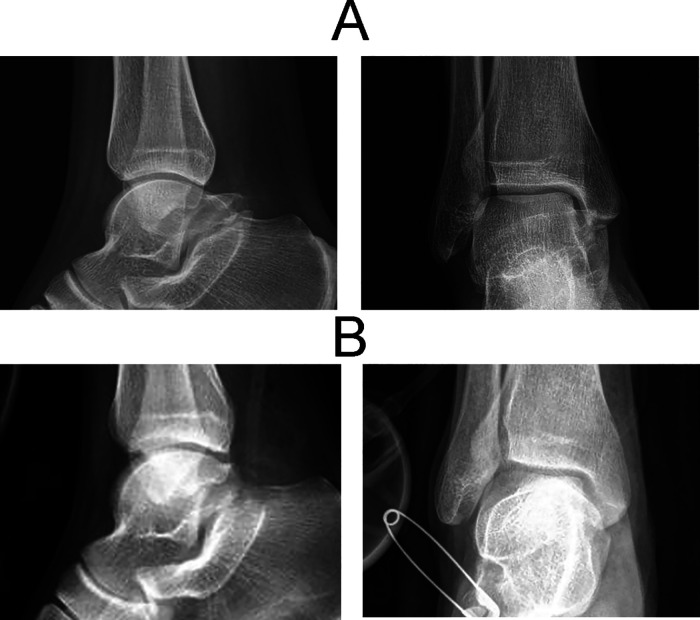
X-rays of TCC pre- (A) and post-operation (B).
